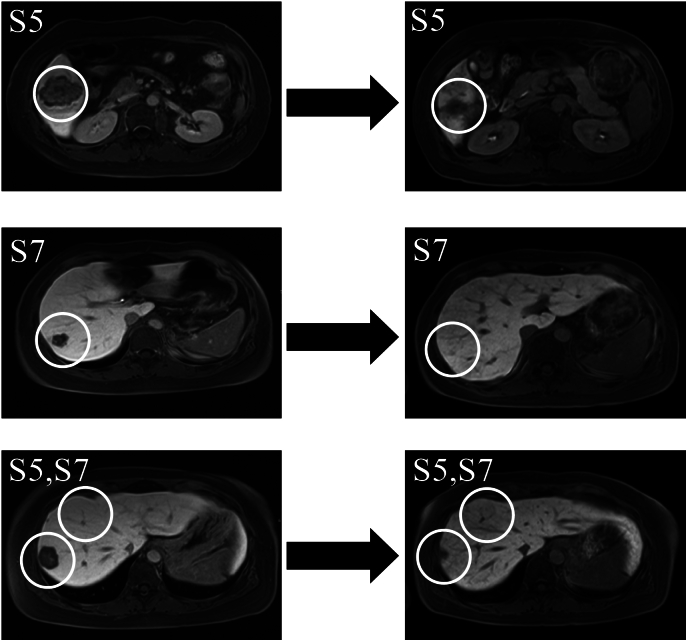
EOB-MRI shows a reduction in S5 and S7 lesions after eight courses of FOLFOX-6 (levofolinic acid, 5-fluorouracil, and oxaliplatin) (white circles). Left column, image taken prior to chemotherapy; right column, image taken post-chemotherapy.
